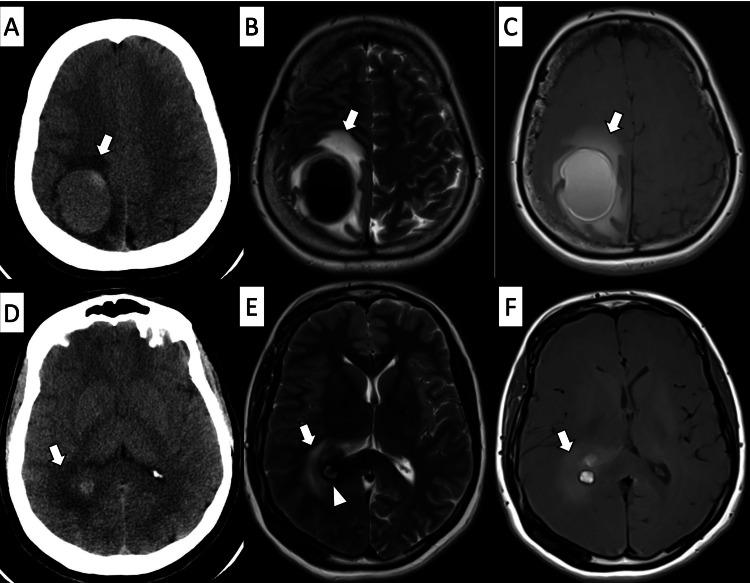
CT and MRI scan showing hyperintense perilesional edema A and D are selective images of an unenhanced CT scan of the brain at the level of the centrum semiovale and posterior horn of the right lateral ventricle, respectively. They exhibit an iso- to hyper-dense, well-defined lesion with surrounding vasogenic edema (arrow) within the right parietal lobe. B and E are T2-weighted images at the same mentioned levels, displaying a hypointense lesion superiorly and hyperintense content inferiorly with a hypointense rim (arrowhead on E) and surrounding hyperintense vasogenic edema (arrow). C and F show the same lesion on T1-weighted images without contrast, demonstrating a gradient of hyperintense content with a hypointense rim and hyperintense perilesional edema (arrow).
